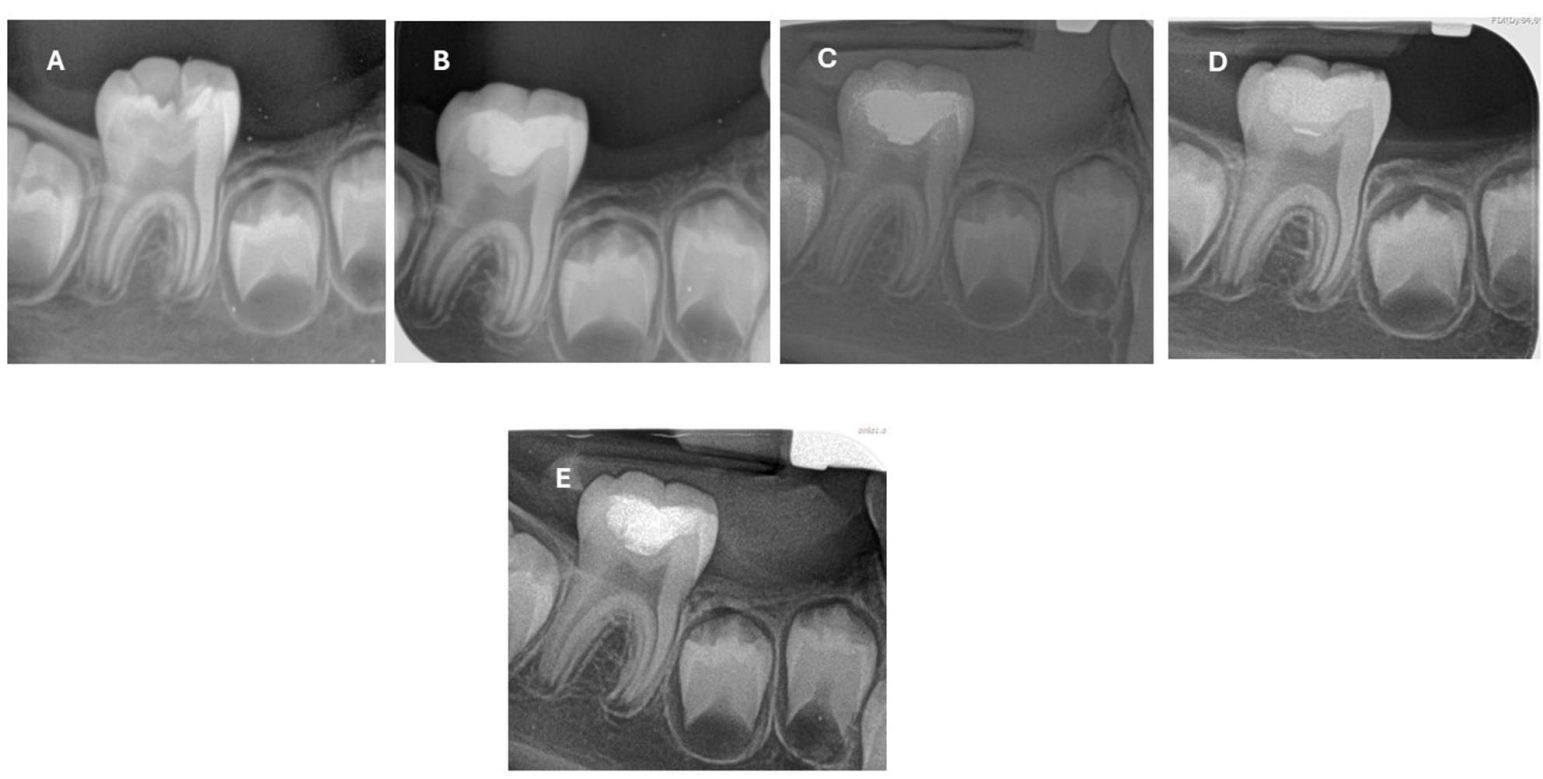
Radiographic follow-up of premixed MTA; (A) preoperative, (B) immediate postoperative, (C) 3-month postoperative, (D) 6-month postoperative, and (E) 12-month postoperative
